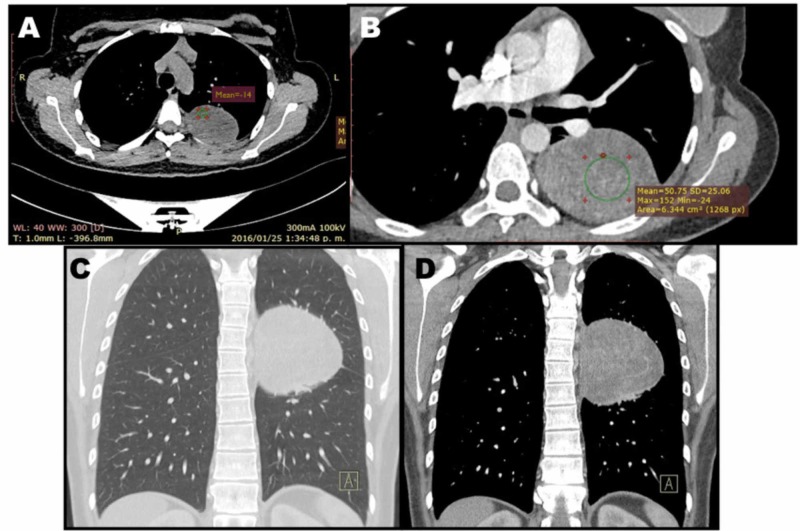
Chest CT scan. A. Axial pre-contrast CT scan of the chest showing a lesion attached to the parietal pleura, heterogeneous with areas of lower density (-14 HU) indicating the presence of macroscopic fat. Adjacent passive atelectasis was seen. B. Axial post-contrast CT scan showing heterogeneous enhancement going from an average of 16 to 55 HU indicating areas of hypervascularity. There is no widening of the neural foramina or origin in the medullary canal indicating the noncontiguity with the dorsal roots. No bone erosions indicating slow growth of benign appearance. No large vessels are irrigating the lesion. C. Coronal reconstruction of the CT scan showing the mass. D. Mediastinal window of the CT scan.
